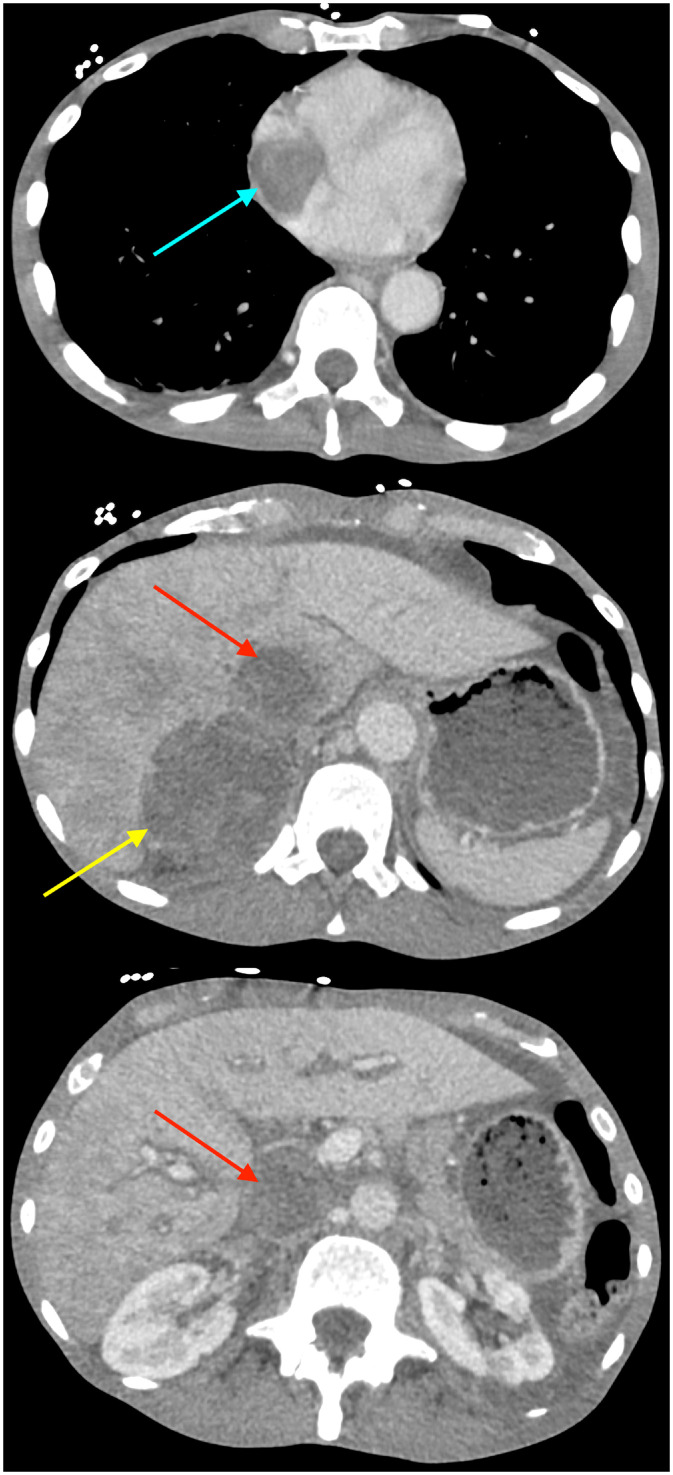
Computed tomography of the abdomen and pelvis with contrast (venous phase) in axial view demonstrates a 7.7 cm right adrenal mass (yellow arrow) with intravascular tumor invasion into the inferior vena cava (red arrow) and right atrium (blue arrow).
